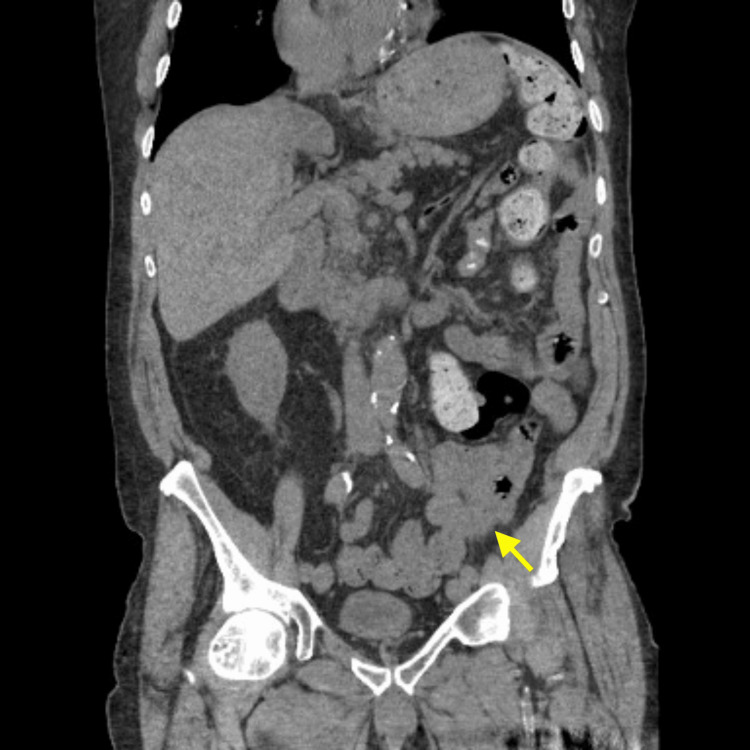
Abdominal/pelvic CT without contrast, coronal section demonstrating redundancy of sigmoid colon with dilatation up to 9 cm, taken three years prior to admission CT, Computed Tomography
